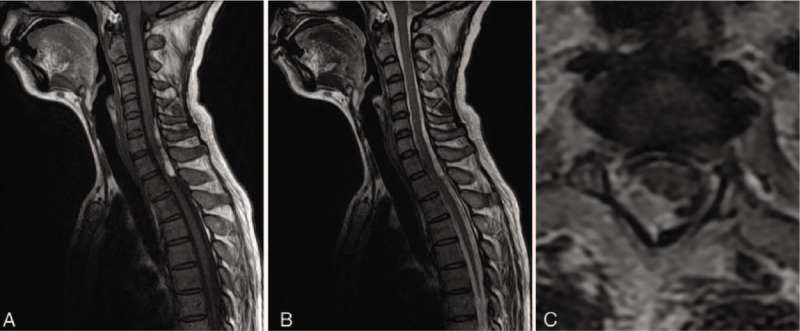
Spinal MRI obtained on day 7 during hospitalization. (A, B) Sagittal spinal MRI showed a subacute subdural hematoma extending from the level of C6 to T1. (C) T2-weighted transversal image at the level of C8 showed the recent hemorrhage in subdural space with no obvious thecal sac compression. MRI = magnetic resonance imaging.
